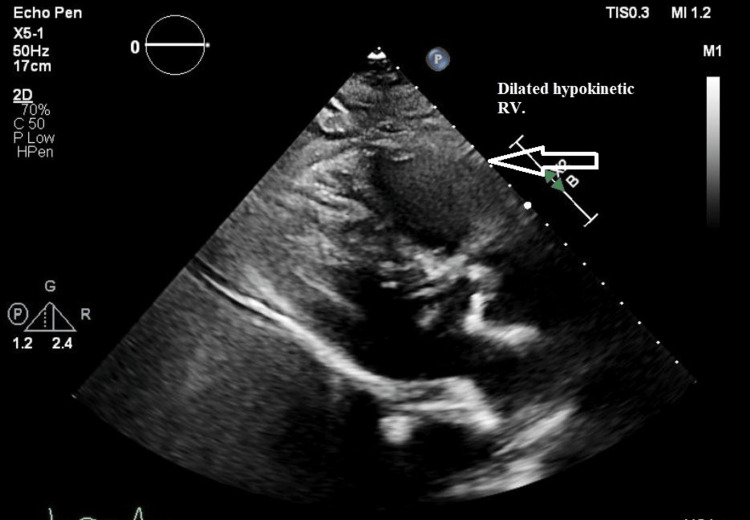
Parasternal short-axis echocardiographic view demonstrating flattening of the interventricular septum (arrow), resulting in a “D-shaped” left ventricle consistent with acute right ventricular pressure overload.
